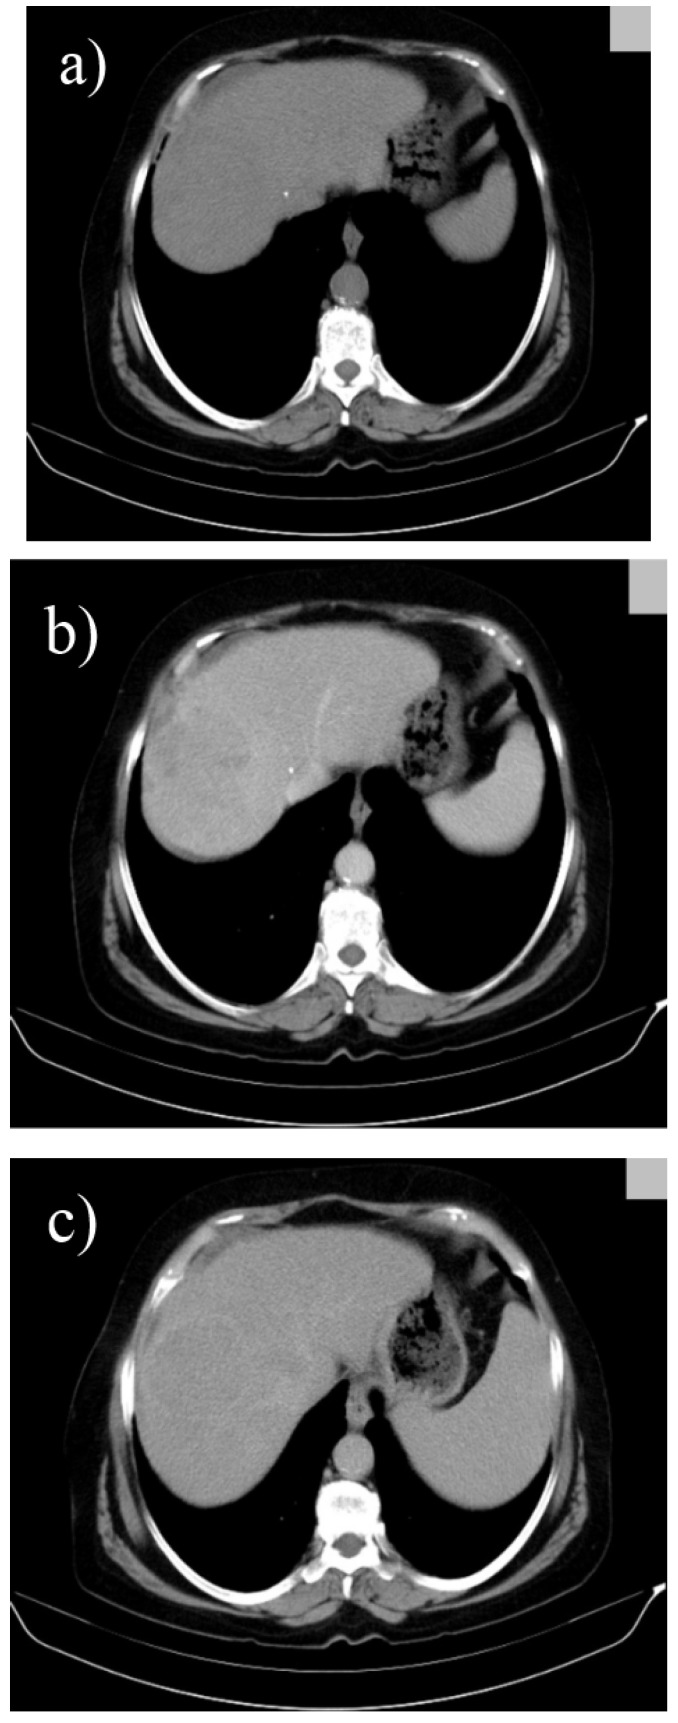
Triphasic multislice CT in a cirrhotic patient showing a well differented HCC in the right lobe with the same density as liver parenchyma in precontrast phase (a), with discrete low density during portal phase (b) and low density with hiperattenuating capsule in the late phase (c)
